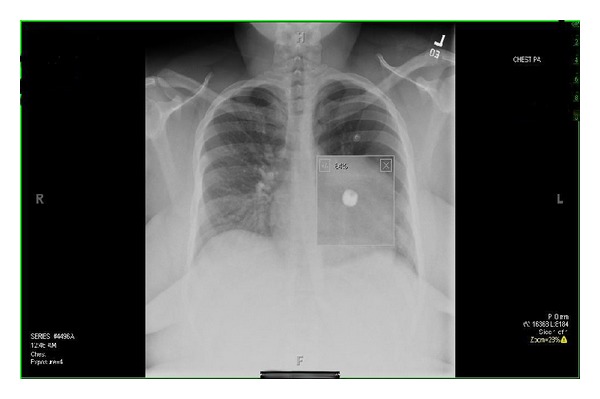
Chest X-ray showing magnified image of the “ball” portion of the tongue piercing in the left main stem bronchus.
